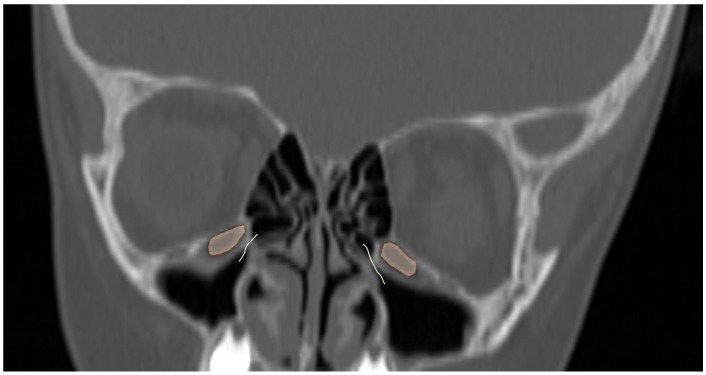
Coronal reformatted CT image of a 6-year-old girl with bilateral Haller cells (orange areas) narrowing the osteomeatal complex (curved lines).
